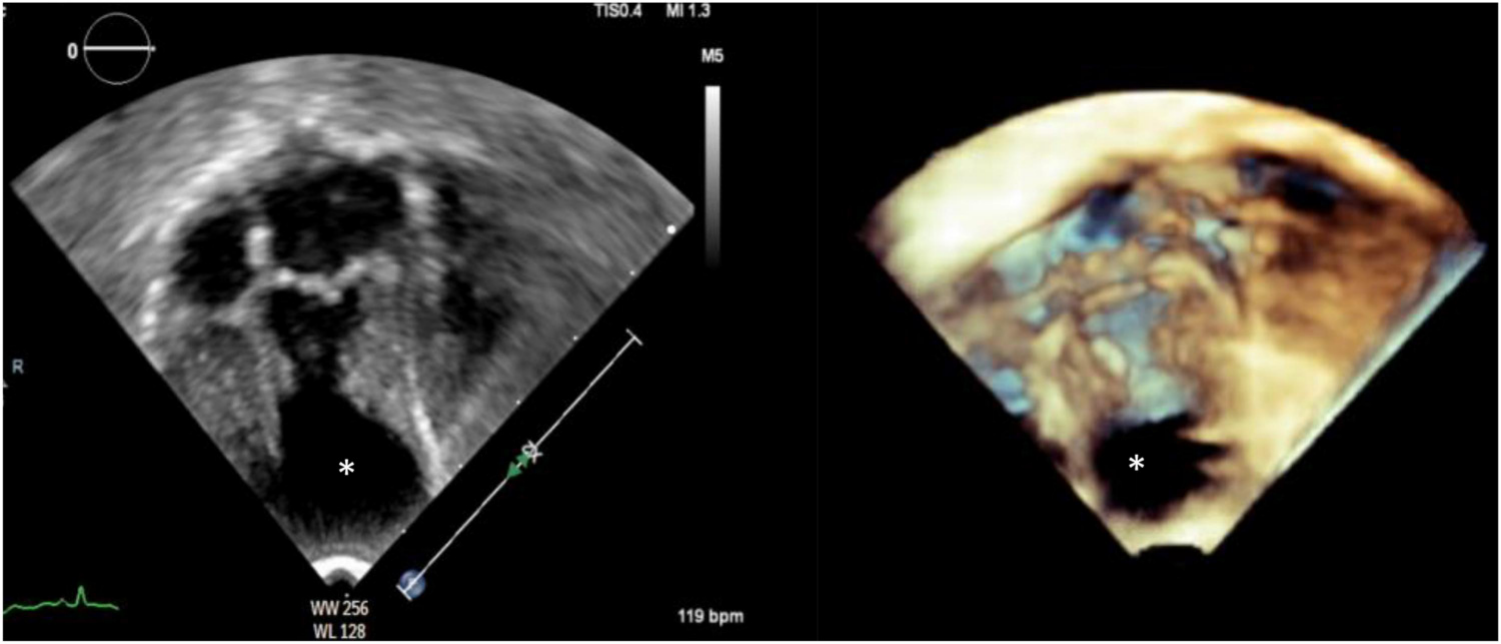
3D echocardiography showing an apical four-chamber view during systole. Dilated apical left ventricular aneurysm (asterisk) while the left ventricle is contracting (asynchrony).
